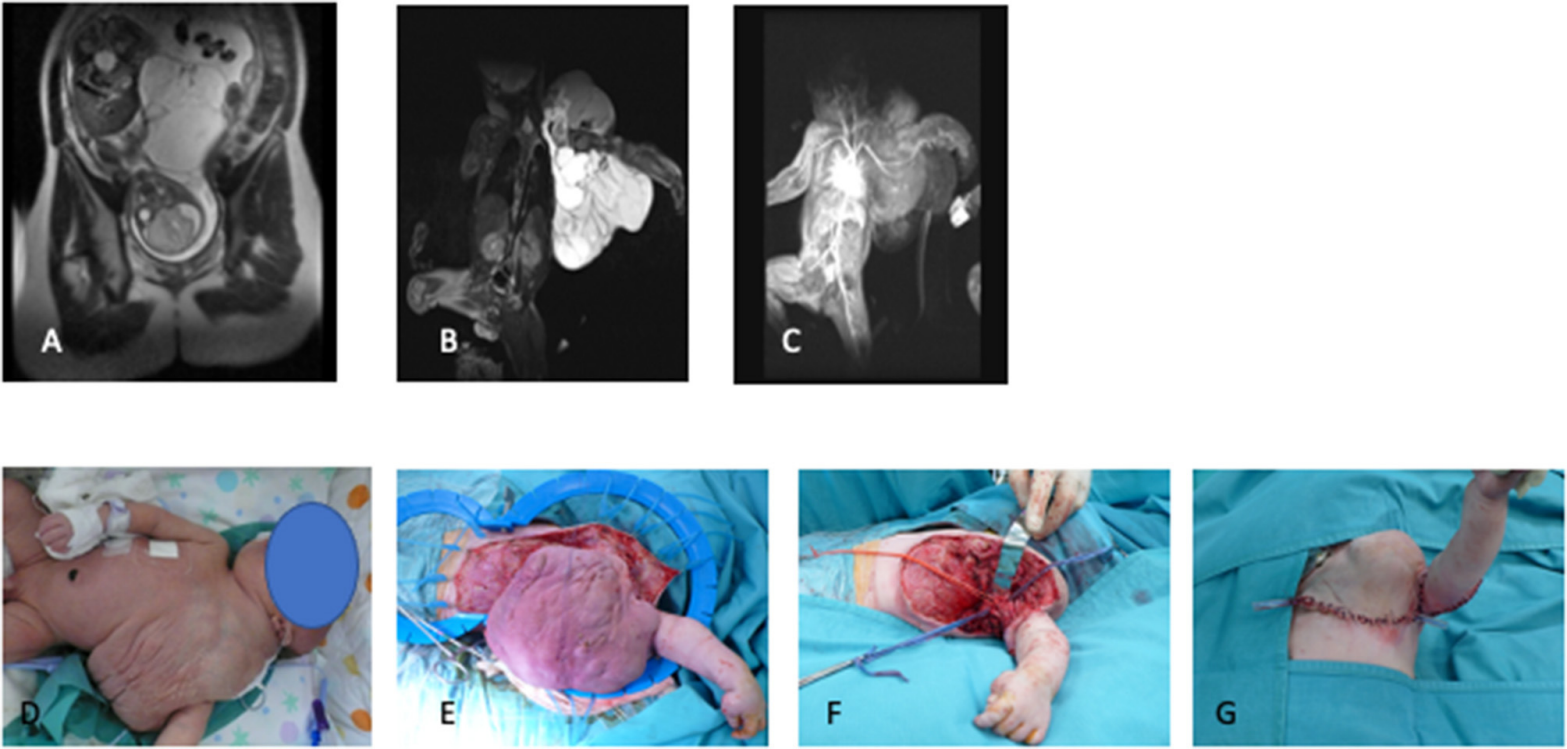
(A) Fetal MRI, (B,C) postnatal T2-weighted MRI sequences, (D) preoperative appearance, (E,F) intraoperative dissection, (G) final surgical reconstruction.
